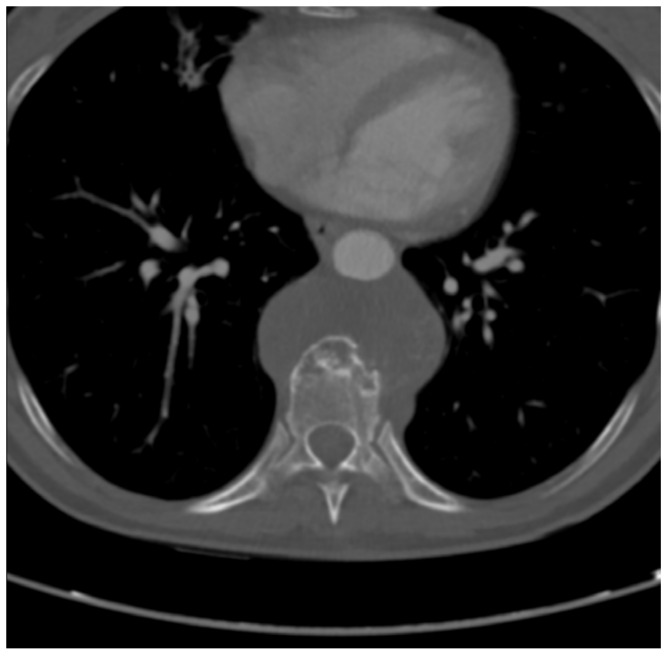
CT scan transverse image crossing T9 shows a lytic, moth-eaten change involving the anterior part of the T9 vertebral body with huge anterior mediastinal soft tissue partially surrounding and displacing the aorta. The transpedicular trocar track for biopsy is visible.
